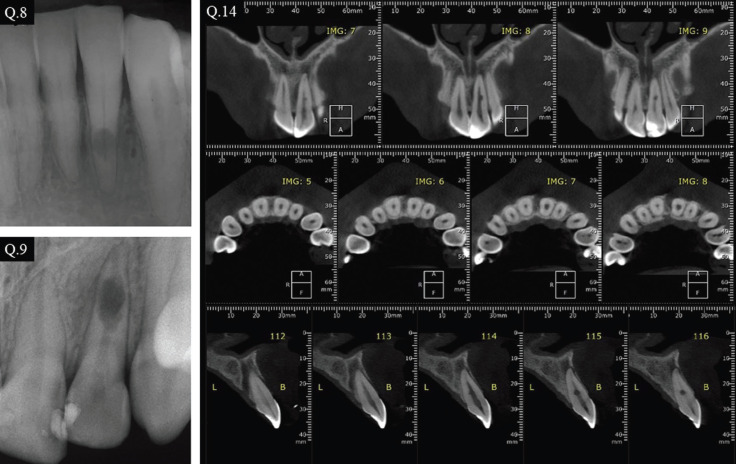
Images of the cases presented in the questionnaire. Q.8: radiograph representing external root resorption; Q.9: radiograph representing internal root resorption; Q.14: CBCT representing internal root resorption.
